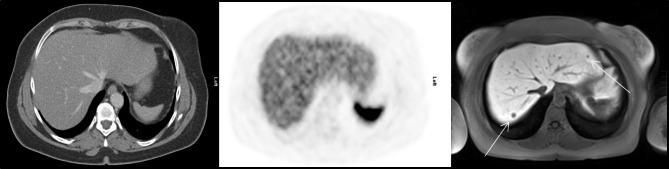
False-negative PET finding in a 52-year-old woman with a NET of the ileum. In the contrast-enhanced CT (left) no suspicious findings of the liver were detected; on PET (middle) no pathological SSR-uptake above the physiological SSR-uptake of liver parenchyma was detected. On hepatobiliary phase MRI (right), small liver metastases with overall low hepatic tumor burden were clearly delineated (arrows)
